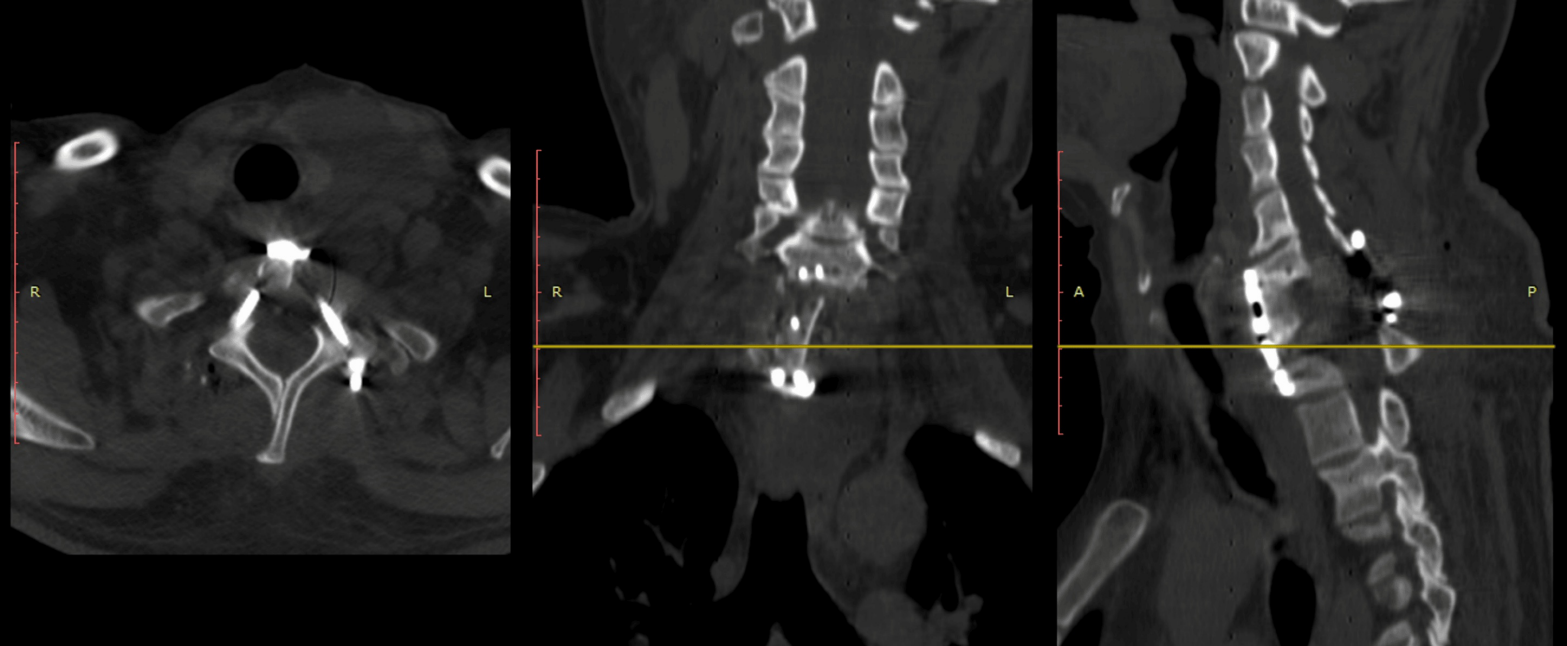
Case 4 non-contrast CT scan of the cervical spine with axial, coronal, and sagittal views of the cervical instrumentation was performed.
